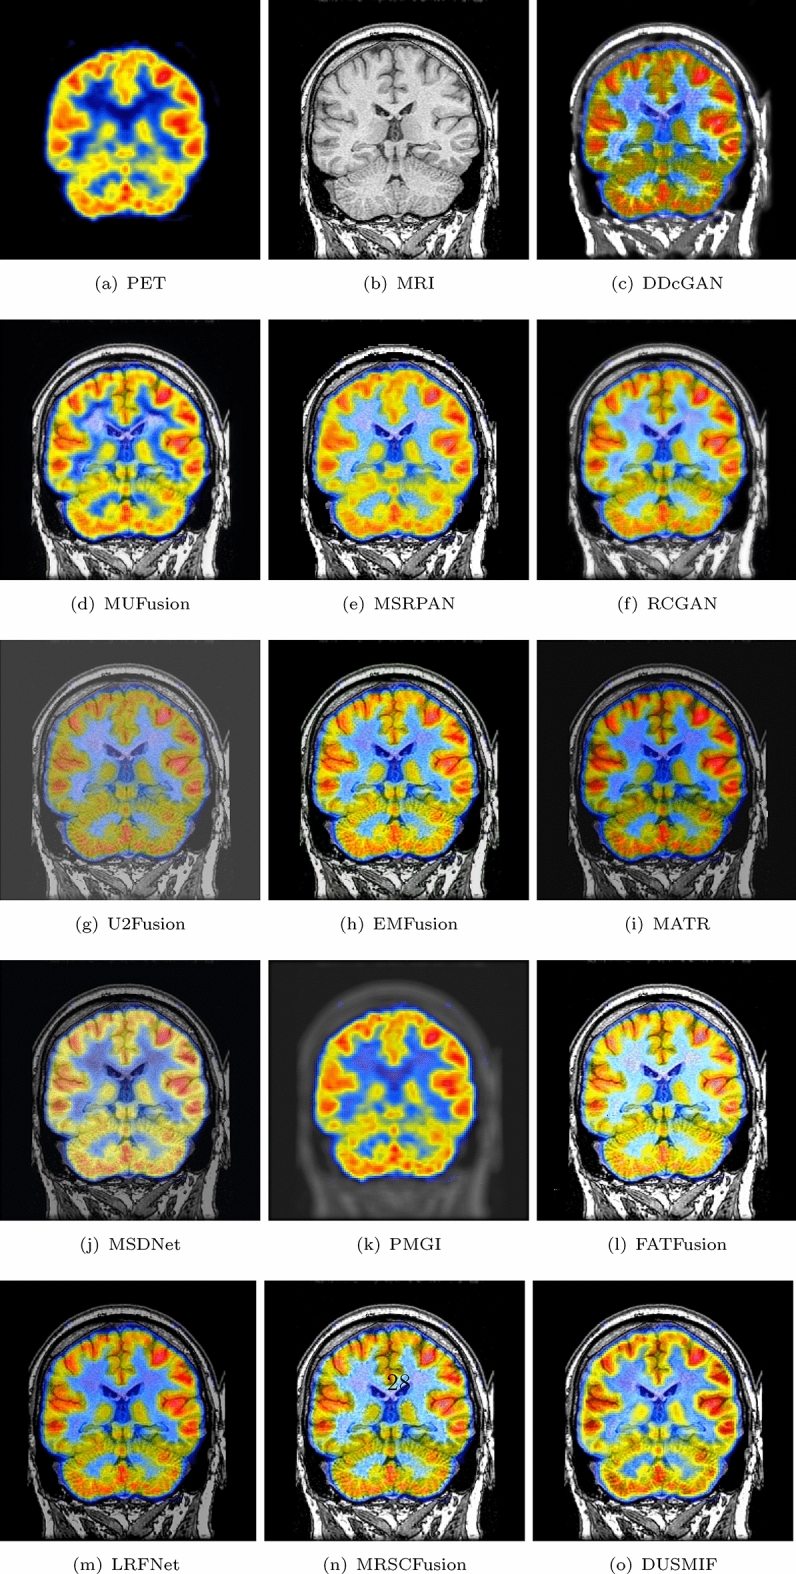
Comparative display of PET-MRI medical image fusion.
